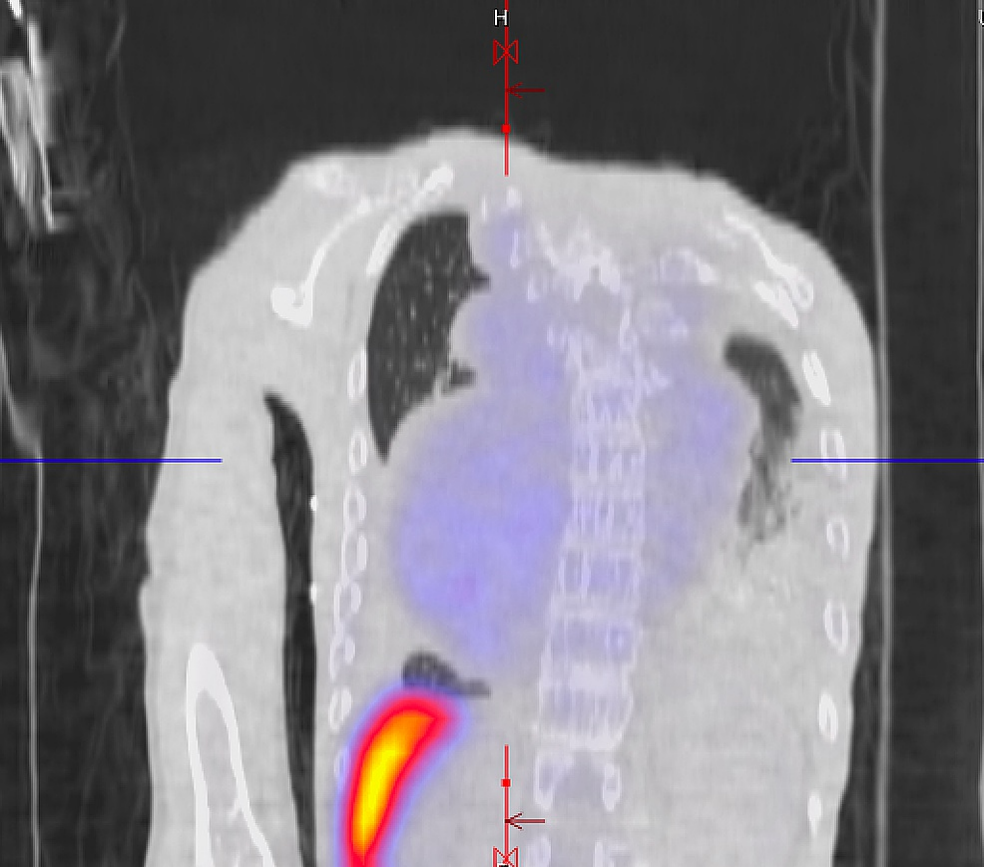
Nuclear Medicine Bone Marrow Scan following the intravenous administration of 15 mCi of 99m technetium-filtered sulfur colloid, and a 20 minute delay, whole body computed tomography images as well as single-photon-emission computed tomography (SPECT/CT) of the chest were performed, where colored areas correspond to uptake of the radiotracer. SPECT/CT images in the coronal plane view depict paraspinal conglomerates at the level of the thoracic spine with associated increased uptake (light blue), suggestive of extramedullary hematopoiesis, compressing the thoracic cavity without involvement of the lung parenchyma, and widespread marrow expansion, with a markedly enhanced liver capsule (red-yellow).
